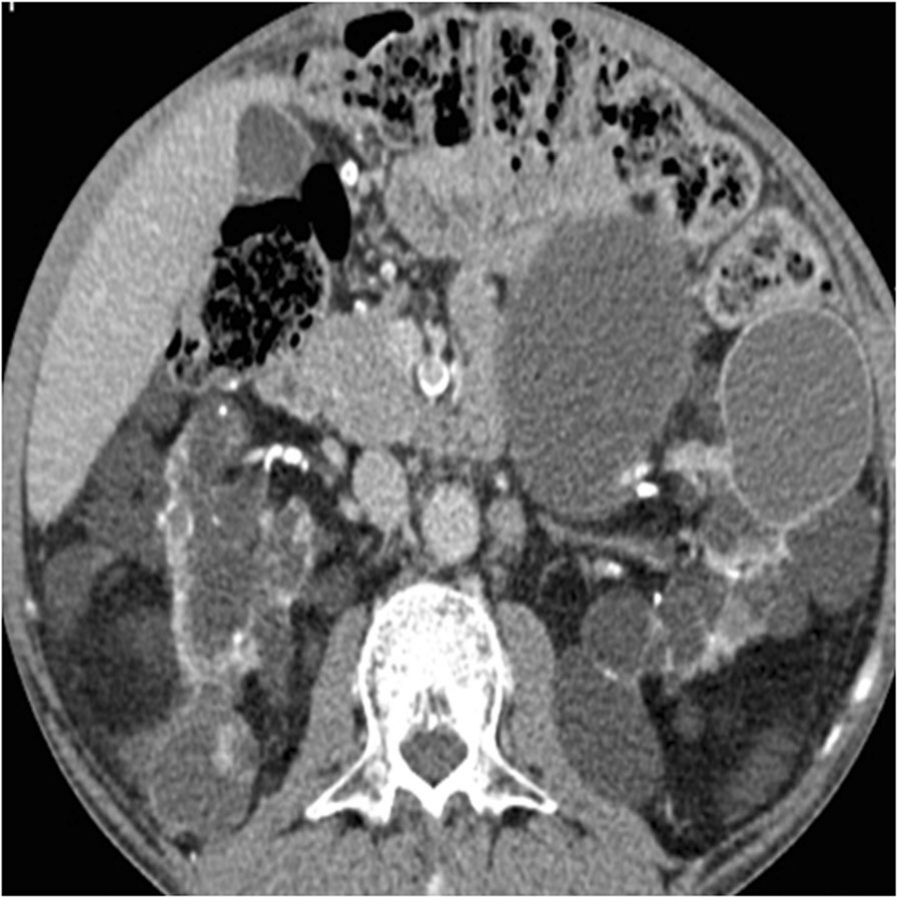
Acquired cystic renal disease. Axial contrast-enhanced CT image shows atrophic kidneys, which contain multiple cysts of variable size
